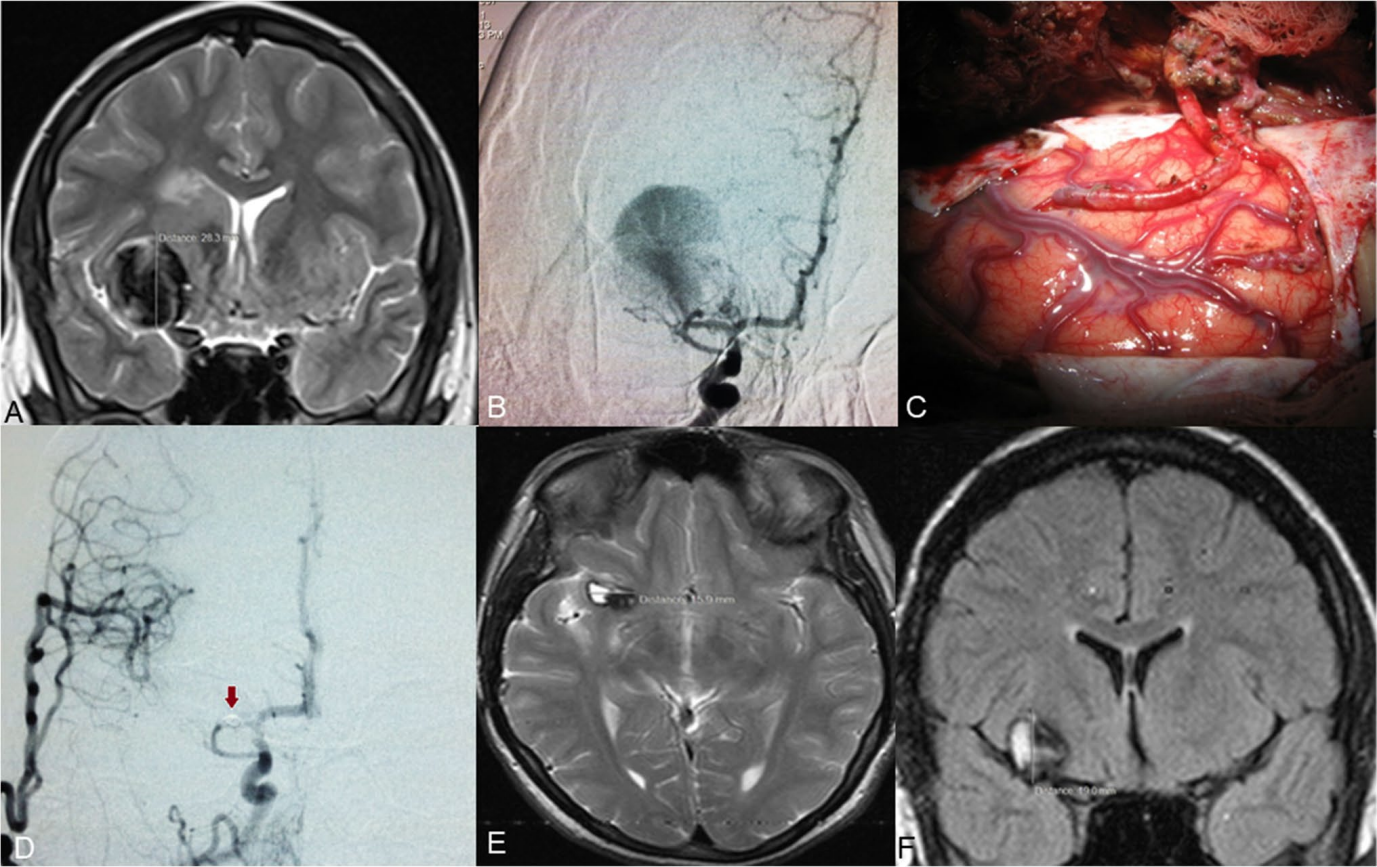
Frontal (A) T2-weighted MRI showing the giant intracranial aneurysm of the MCA. DSA from the right ICA (B) shows the aneurysm of the M1 segment of the MCA. Intraoperative view showing the STA-MCA (M4 segment) anastomosis (C). DSA from right CCA (D) demonstrates the total occlusion of the right M1 segment aneurysm and the patency of the double-barrel STA-MCA bypass. Axial (E) T2-weighted, and frontal (F) brain T1-weighted MRI performed after 9 months, showing the reduction in the size of the aneurysm of the MCA
